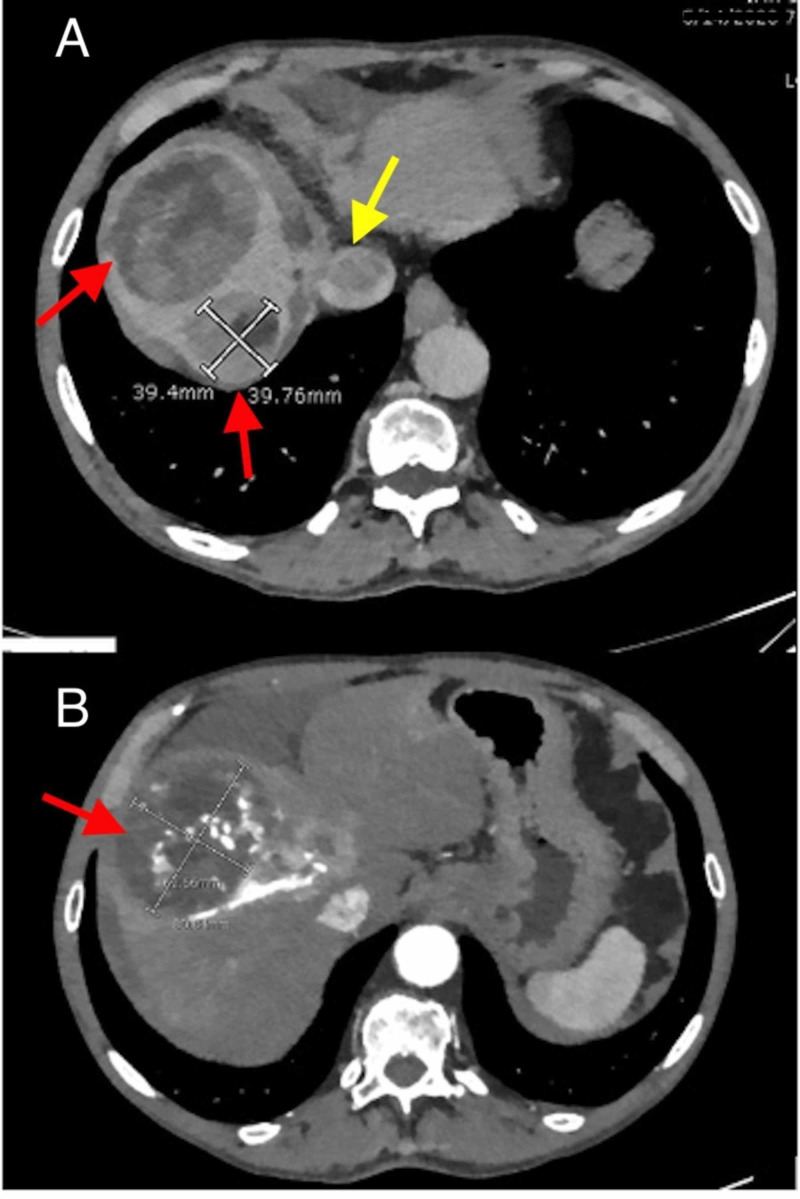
Abdominal CT scan with contrast. A: two hepatic observations consistent with HCC (red arrows), IVC tumor thrombus (yellow arrow). B: large hepatic observation (red arrow). HCC, hepatocellular carcinoma; IVC, inferior vena cava
